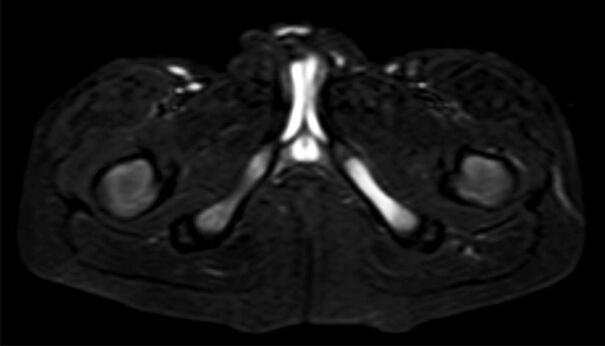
T2-weighted MRI scan with a left-sided VND suspicious of osteomyelitis in a 7-year-old male patient.
